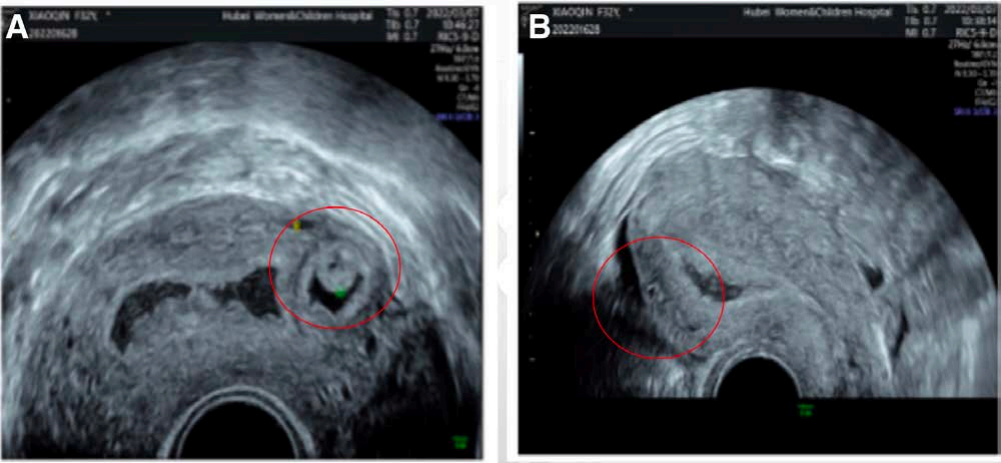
Transvaginal ultrasonography showed a gestational sac inside in the myometrium below the right uterine horn with blood flow signals around, and there was a small passage connecting the intramural gestational sac with the uterine cavity.
